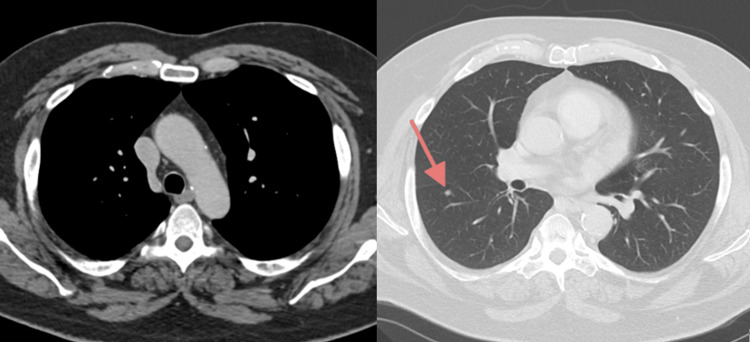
Axial chest CT images in soft tissue window (left) and lung window (right) There is no evidence of mediastinal mass or thymoma. An incidental 5mm pulmonary nodule noted in the right lower lung lobe (right image, arrow), for which surveillance imaging was recommended. CT, computed tomography
